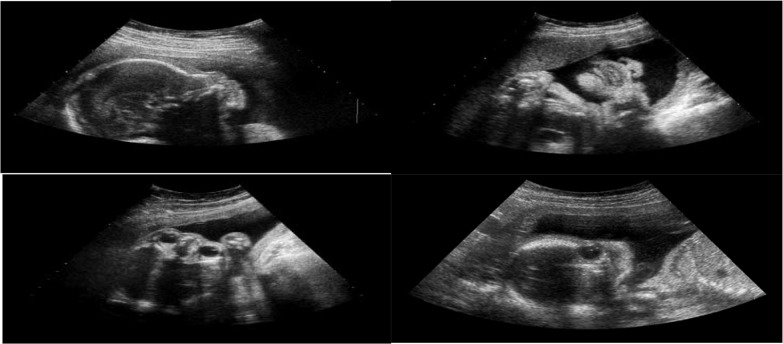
Different planes of fetal ultrasound (US) image samples for training (left upper is axial plane, right upper is coronal plane, left bottom is sagittal plane, and right bottom is non-fetal facial standard plane (FFSP)).
